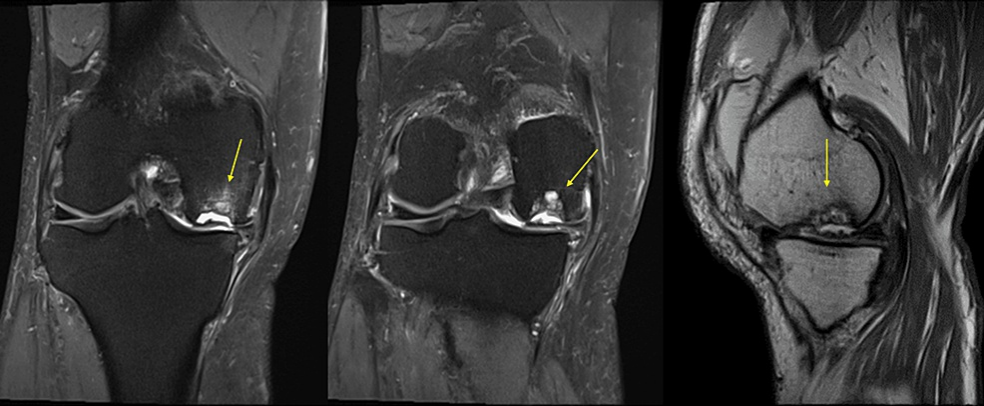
Preoperative MRI The arrows indicate bone and cartilage defects with bone marrow edema.
